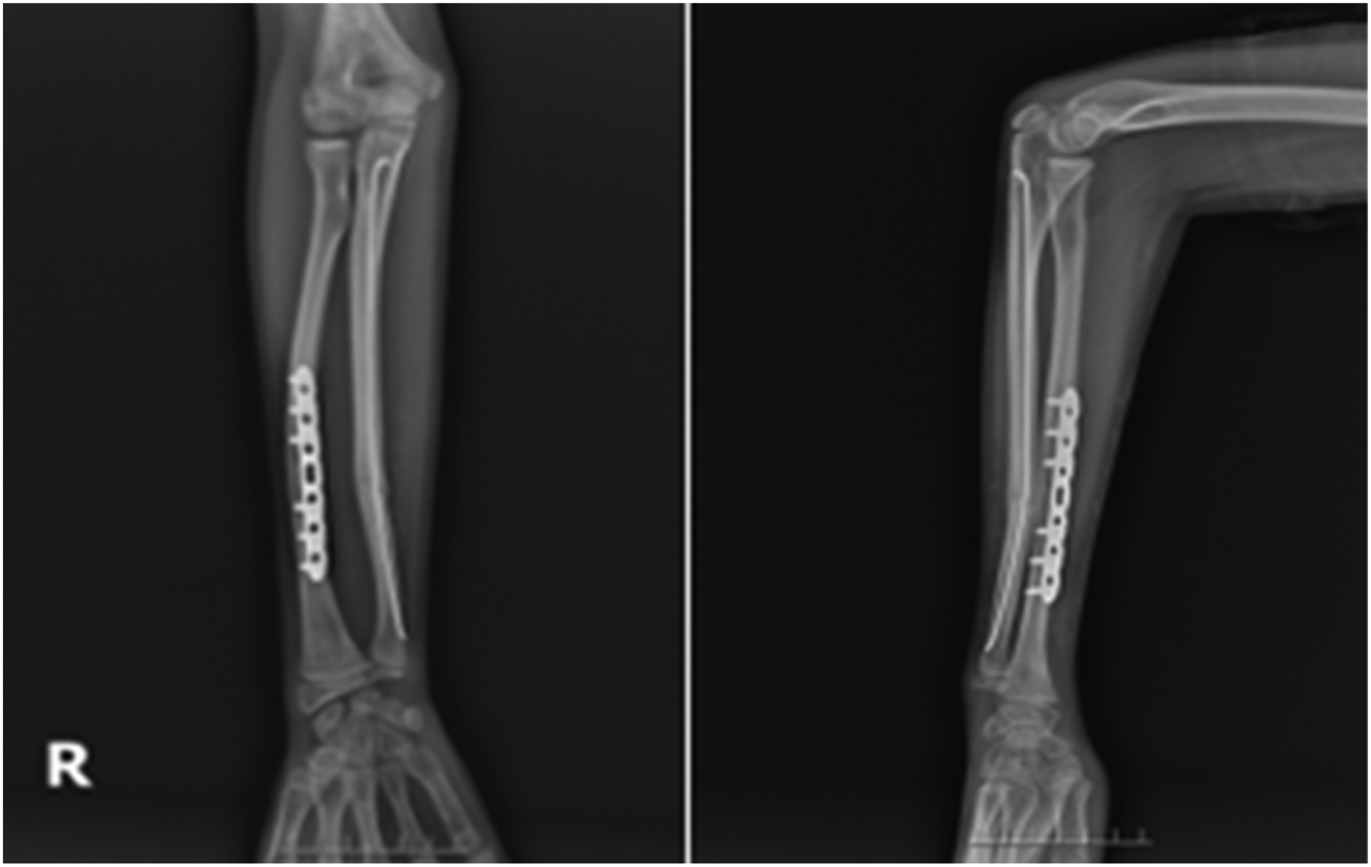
Postoperative x-ray at 5 months.
